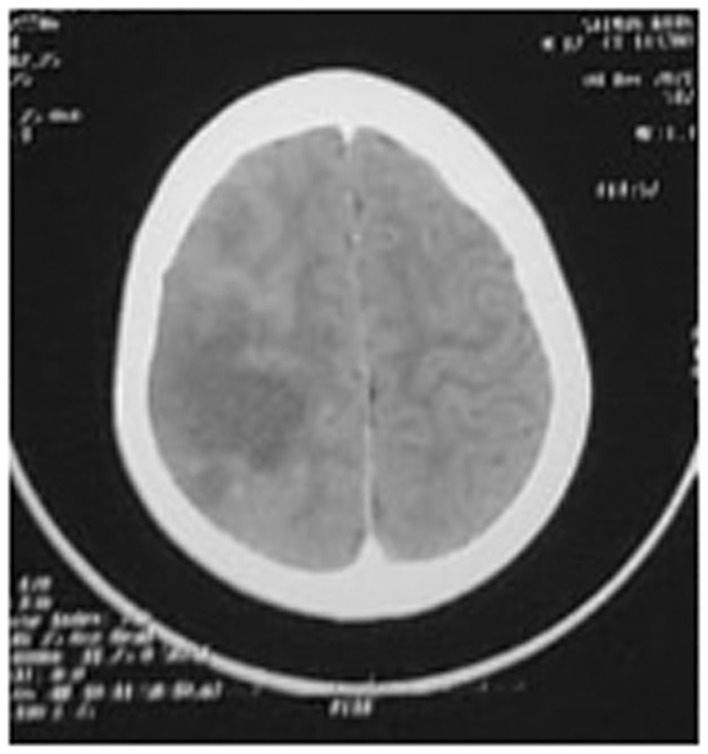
CT head showing hypodense area on right temporo‐parietal region.
